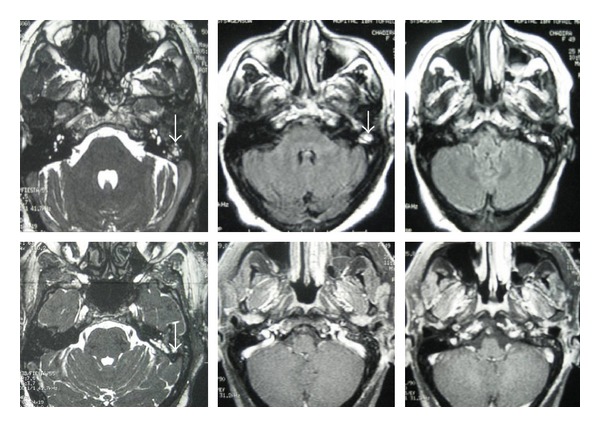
MRI showing a nodular lesion in intermediate signal T1 and Hyper signal T2 with enhancement after gadolinium injection.
